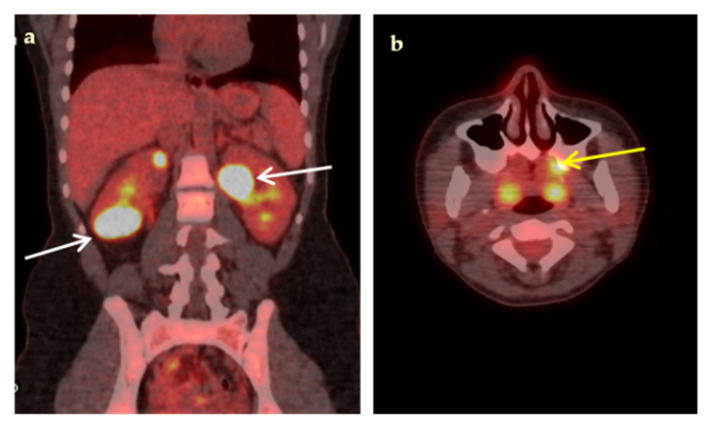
An 18-year-old woman presented with a 3-week fever, elevated inflammatory blood markers (ESR 77 mm/h, CRP 9.3 mg/dL) and an abdominal CT scan suggestive of possible renal abscesses. Coronal fused ^18^F-FDG-PET/CT image (a), demonstrated multiple hypodense, highly hypermetabolic lesions in both kidneys, the largest (white arrows) in the lower pole of the right kidney (SUVmax 35.3) and in the upper pole of the left kidney (SUVmax 34.4), compatible with renal abscesses, confirmed by biopsy. Transaxial fused ^18^F-FDG-PET/CT image (b) demonstrated high ^18^F-FDG paradental uptake in the left maxilla (yellow arrow) raising concern for hematogenous spread of dental infection. Complementary focused interrogation revealed a history of a painful, undertreated dental condition of the left maxilla preceding fever.
